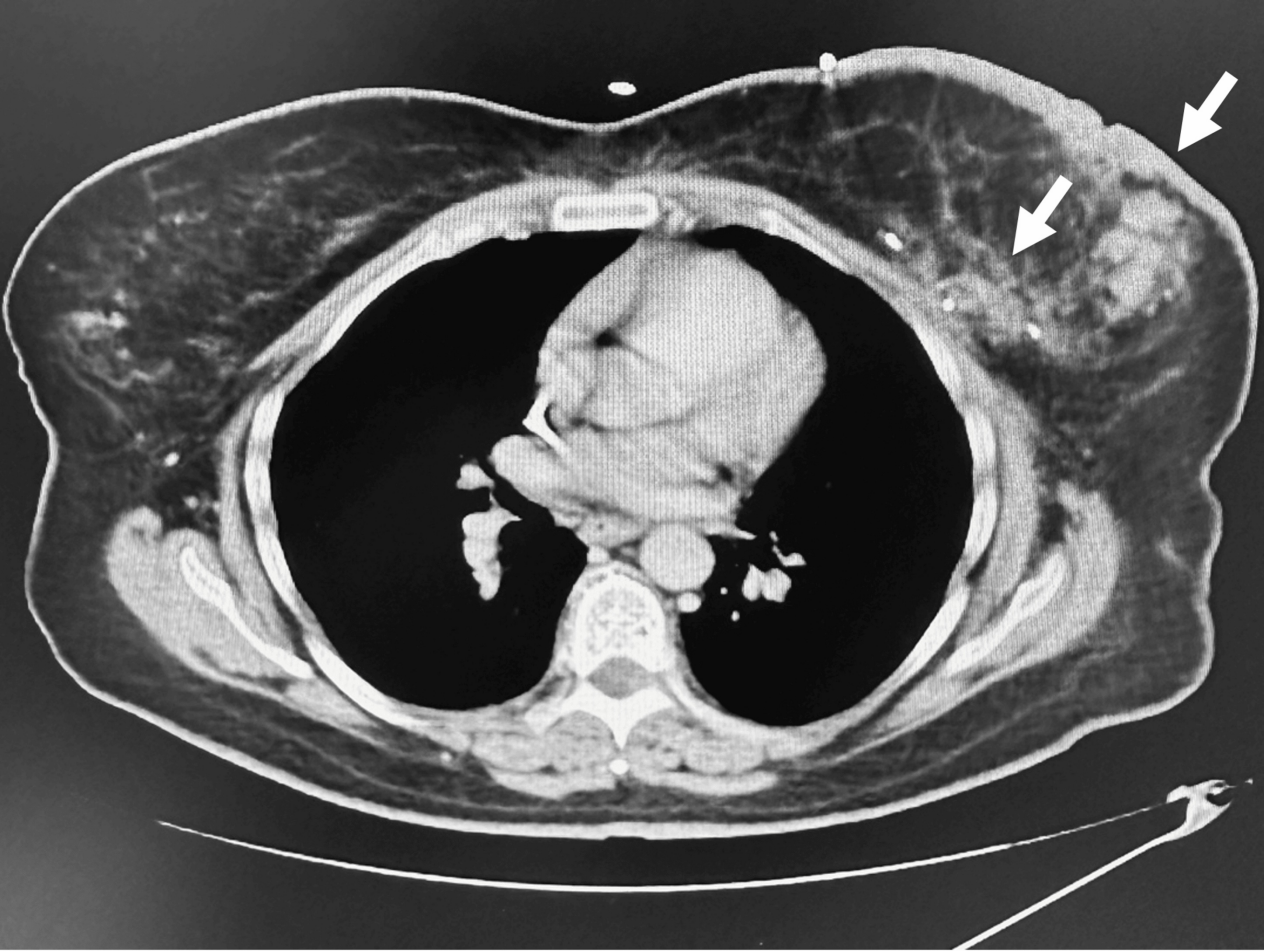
CT of the chest shows left breast thickening with multiple soft tissue metastases.
